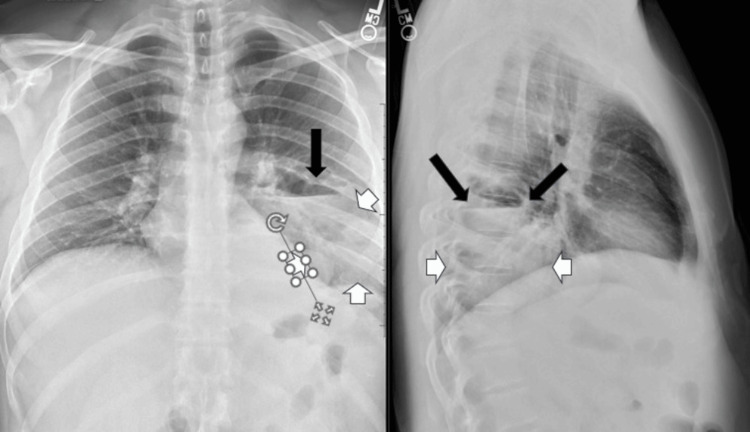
Admission chest X-ray This image shows posterior-anterior (PA) and lateral views of the admission chest X-ray. There is confluent opacity in the left lower lobe posterior thorax (short white arrows) with sharp air-fluid levels (long black arrows).
